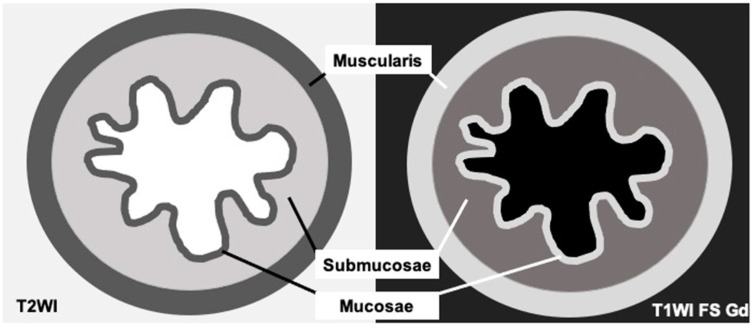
Scheme of esophagus wall appearance on MRI. Sequence T2WI: the mucosa is thin and hypointense, the submucosa is the most visible layer and is hyperintense, while the muscularis propria is the third visible layer and appears hypointense. Sequence T1WI FS at 3 min post-gadolinium injection: the mucosa is thin and hyperintense, the submucosa is hypointense, and the muscularis propria is hyperintense due to enhancement. WI: weighted imaging; FS: fat sat; and Gd: gadolinium.
